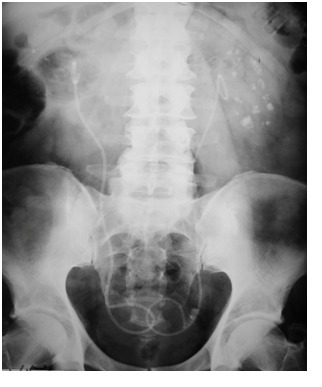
Post-operative KUB X-rays in a patient with bilateral MSK and nephrocalcinosis: 2 procedures on the right side and one on the left
